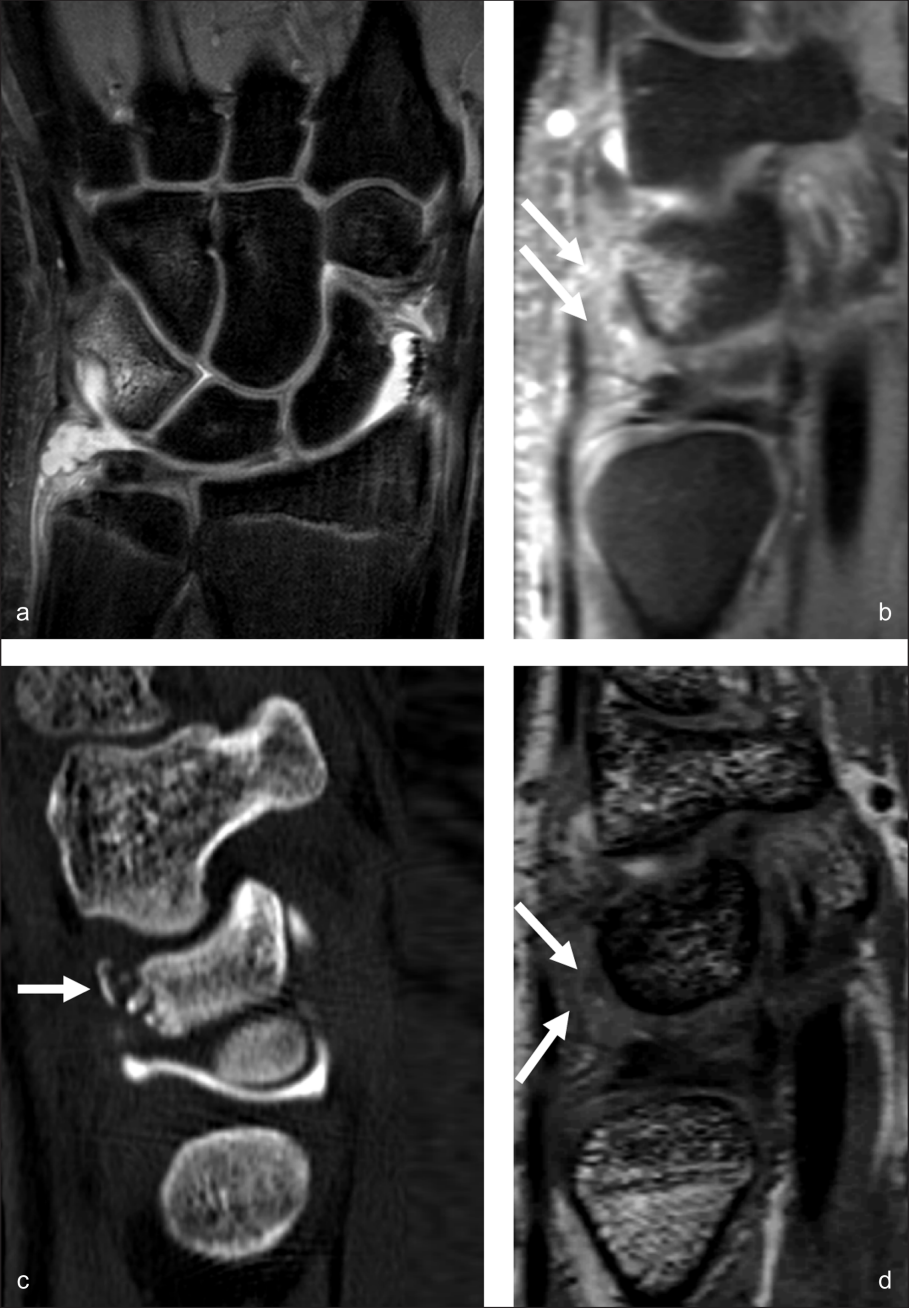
Triquetrum occult fracture with synovitis in the prestyloid recess and traumatic bone avulsion with subacute edematous infiltration of the dorsal capsular ligaments. MR arthrography performed 12 days after a fall on an outstrechted hand allows detection of bone marrow edema in the dorsal part of the triquetrum on coronal proton-density fat-saturated images (a) and sagittal 0.4mm reconstruction from a 3D proton-density fat-saturated sequence (b), as well as edematous infiltration of the surrounding dorsal soft tissues (arrows on b). The dorsally avulsed bony fragments are better seen on CT (arrow on c). The dorsal capsular infiltration (mostly the extrinsic dorsal radiotriquetral ligament) with low to intermediate signal intensity is also depicted on the sagittal reconstruction from the 3D DESS sequence (arrows on d).
